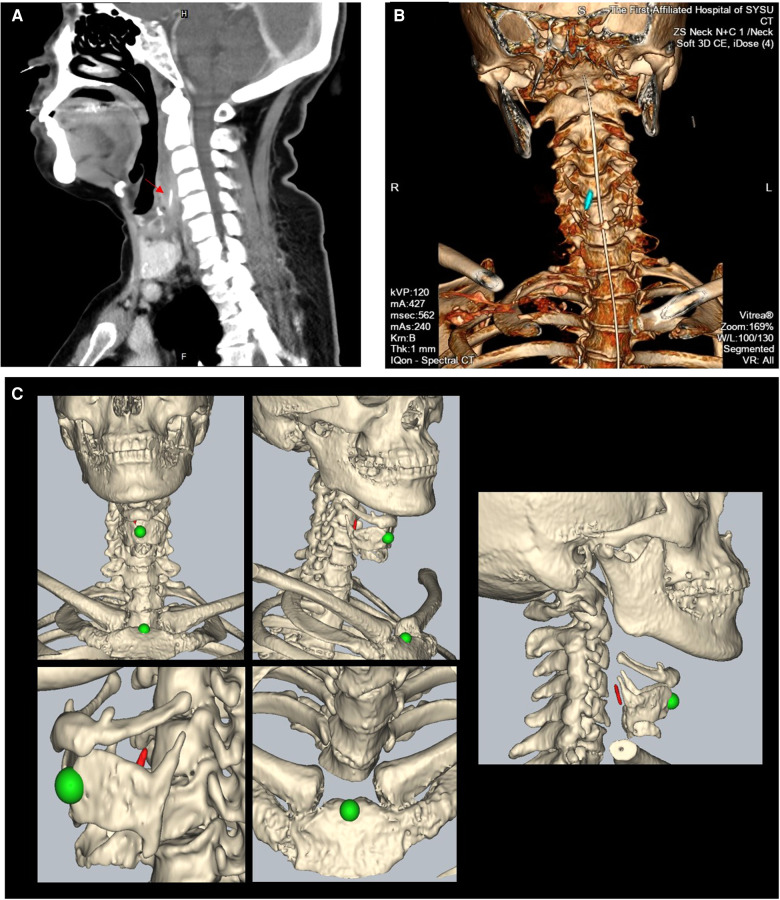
(A, B) Sagittal CT image and three-dimensional construction image of the patient after direct laryngoscopy ((A): the red arrow points to the foreign body); (C) representative images of intraoperative navigation registration: head and face contour scanning, combined with a facial marker, was used for registration, with an average registration accuracy not greater than 1.0 mm. After the model registration is completed, the patient's body position is further adjusted according to the positioning of the superior thyroid notch and the manubrium sterni (green) in the navigation model, so that the neck position from the navigation model matches the actual position of the patient’s neck.
